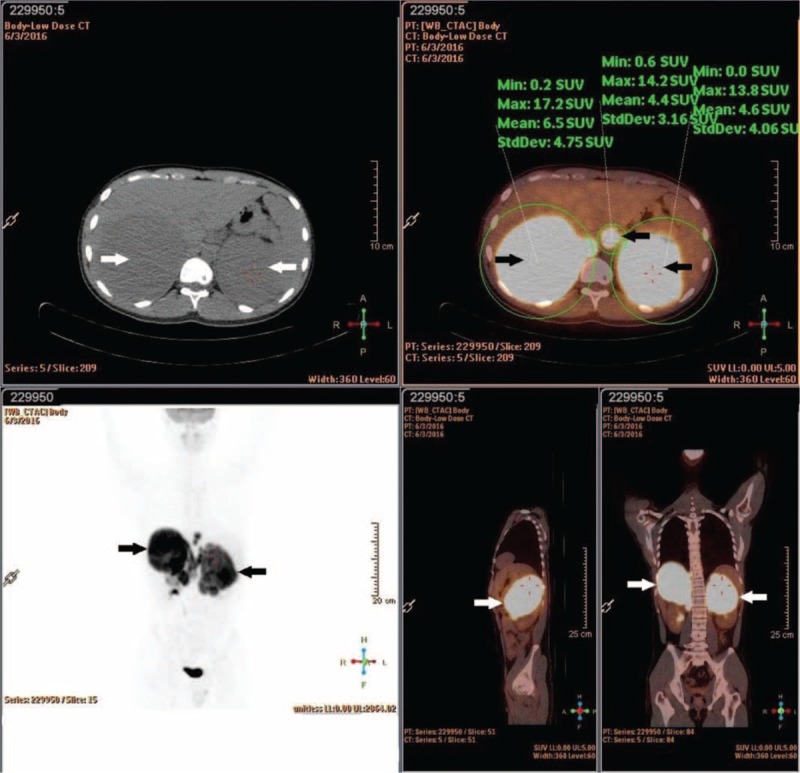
Abdominal CT showed bilateral adrenal masses (top left). ^18^F-fluorodeoxyglucose positron emission tomography (18-FDG/PET) scan (bottom left) and two fusion PET/CT images (top right and bottom right) showed increased FDG uptake in the bilateral adrenal glands and retroperitoneal lymph node. CT = computed tomography, 18-FDG = fluorine-18-fluorodeoxyglucose, PET = position emission tomography.
